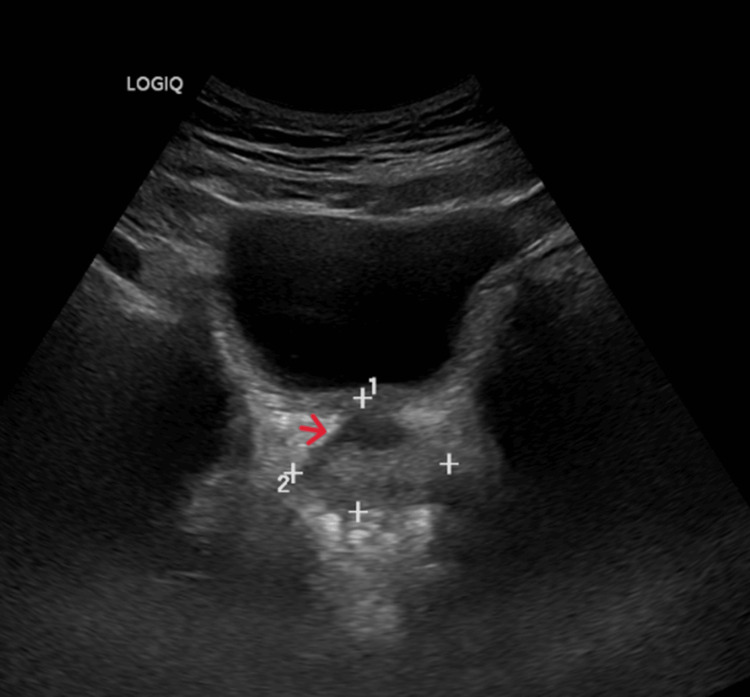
Repeat ultrasound of the abdomen showing no residual prostatic abscess
